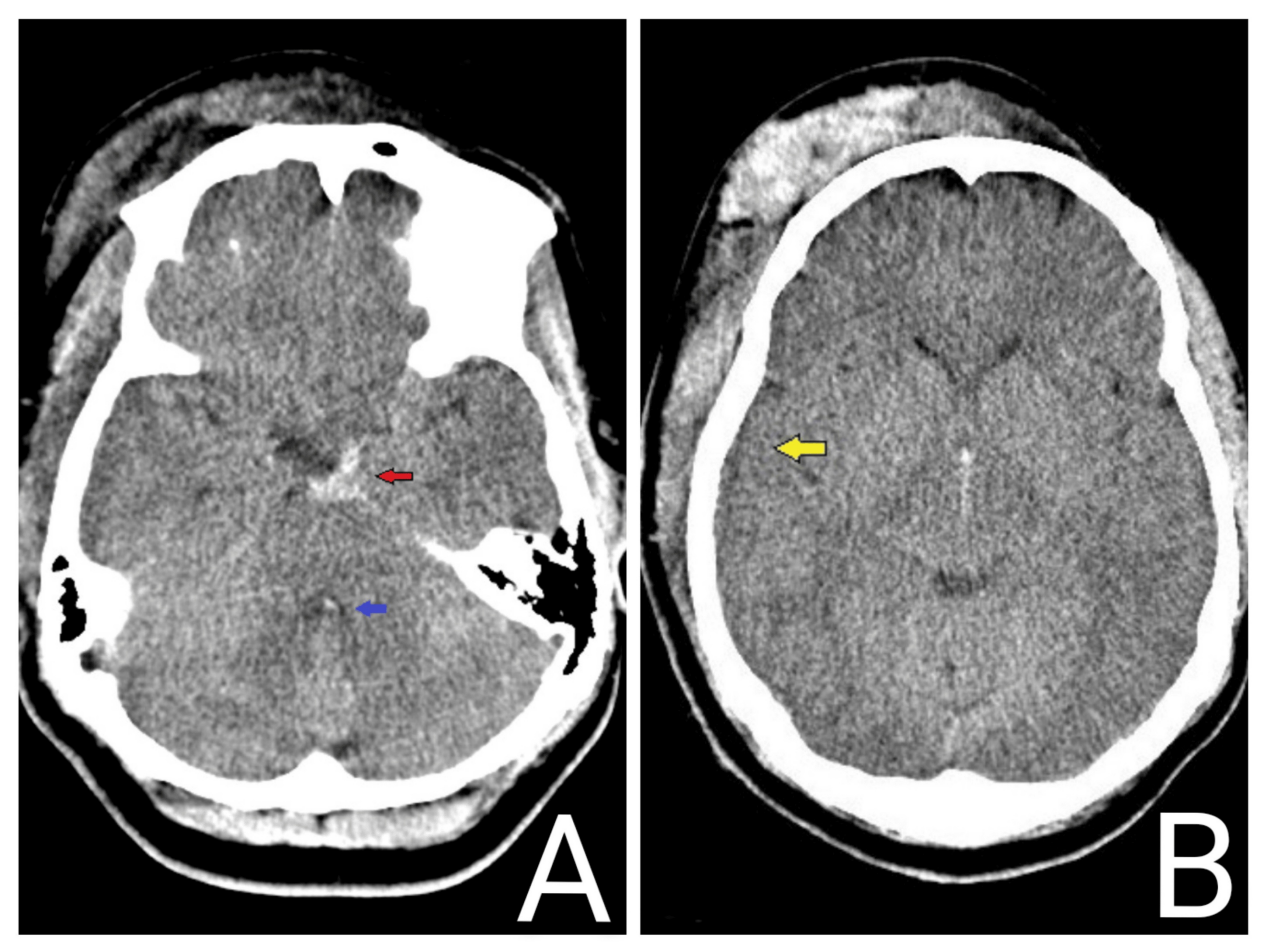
CT head of patient 1 Axial non-contrast CT of the head. (A) Subarachnoid hemorrhage (red arrow) and effacement of the fourth ventricle (blue arrow). (B) Sulci effacement (yellow arrow) and diffuse cerebral edema. On neurological examination, the patient presented with eyes closed to pain, not following commands, pupils 3 mm and minimally reactive bilaterally, localization in bilateral upper extremities, and withdrawal in bilateral lower extremities. CT: computed tomography
